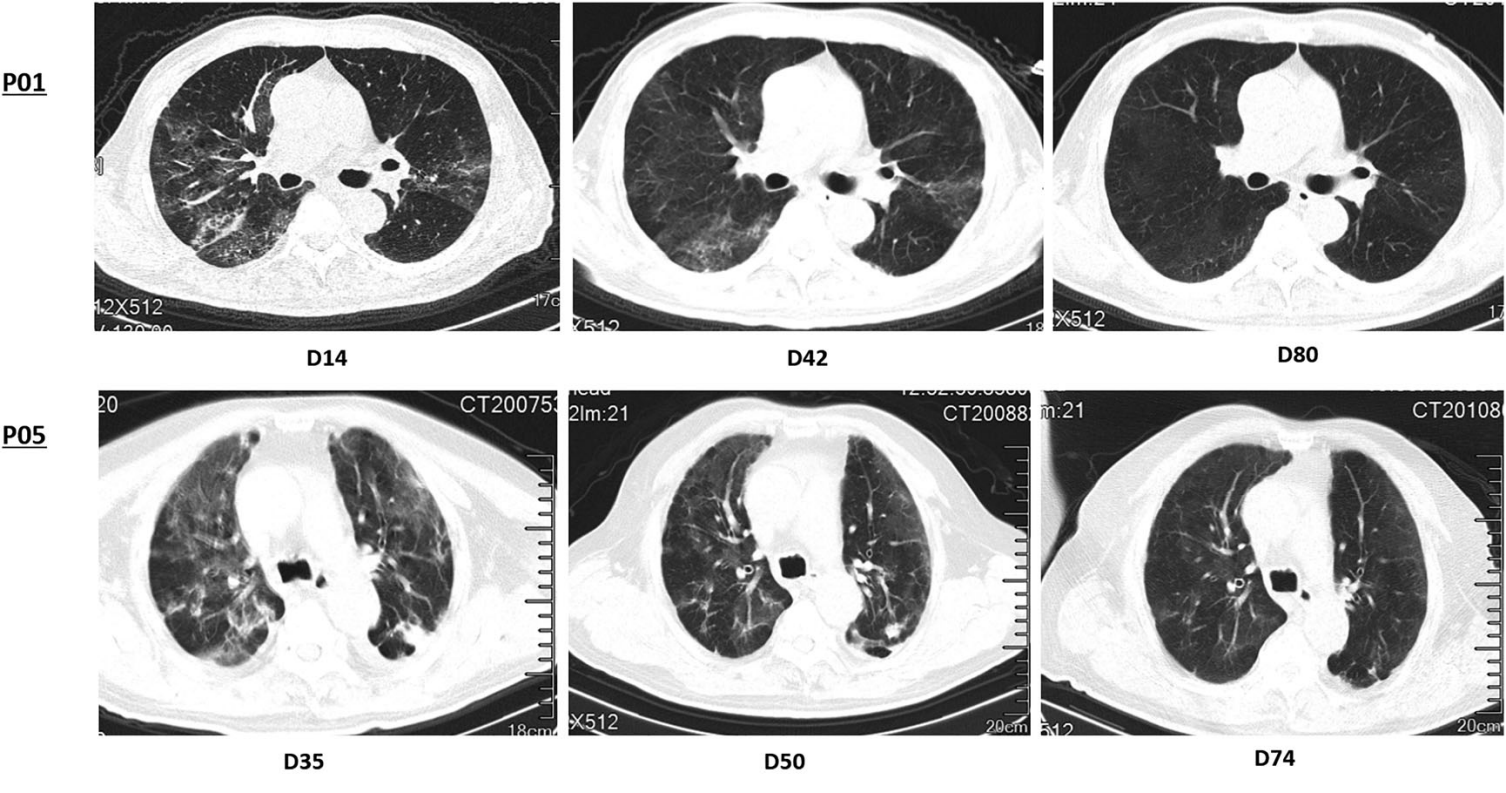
Series of chest CT presentation in patients with prolonged positive PCR results. Both patients did not present with any clinical symptoms. P01 showed typical changes of fully recovery, and P05 showed partial improvement at the time of evaluation.
